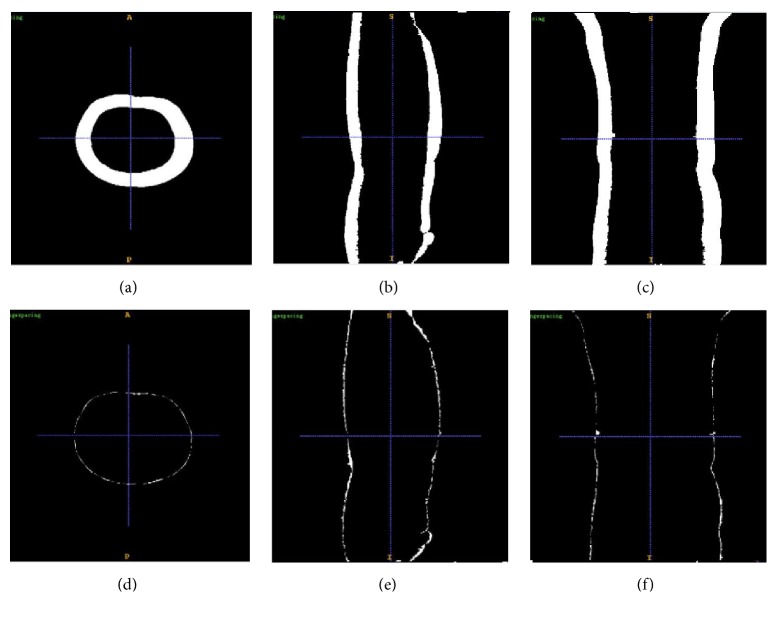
An example of the subtraction of PET and CT feature image sequences. (a), (b), and (c) represent the subtraction before registration and (d), (e), and (f) represent the subtraction after registration. (a) and (d) are along the transverse plane, (b) and (e) are along the sagittal plane, and (c) and (f) images are along the coronal plane.
